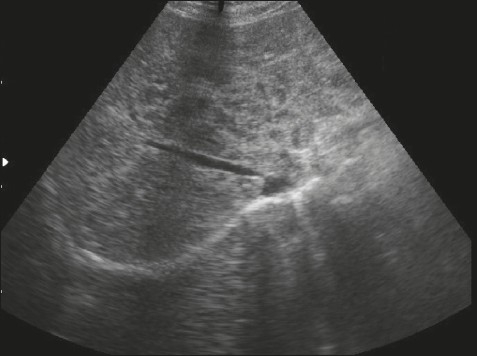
Ultrasound of the liver shows heterogenous echogenicity.
